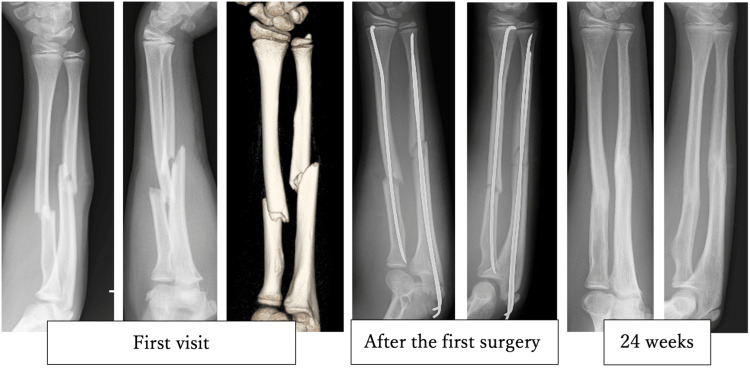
The initial adolescent male, aged 14 X-rays for the first visit, after the first surgery and 24 weeks. The patient suffered fractures in both the radius and ulna bones of the forearm, which were then surgically treated, with a Kirschner wire (K-wire) utilized for stabilization. This wire was removed 14 weeks post-surgery, and by the 24th week, the bones had fused.
